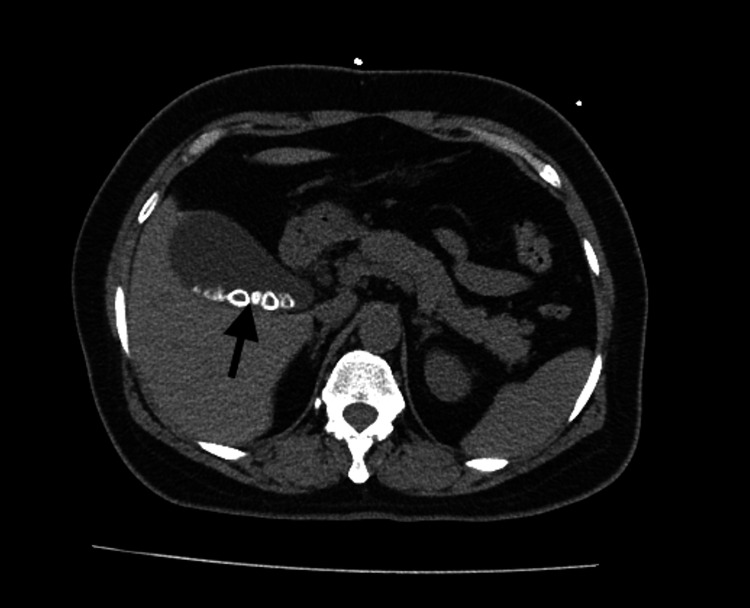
CT abdomen and pelvis without contrast. Significant for a non-distended GB, numerous radiopaque gallstones (black arrow), no wall thickening or pericholecystic fluid, and no intra- or extra-hepatic biliary duct dilation. GB, gallbladder
